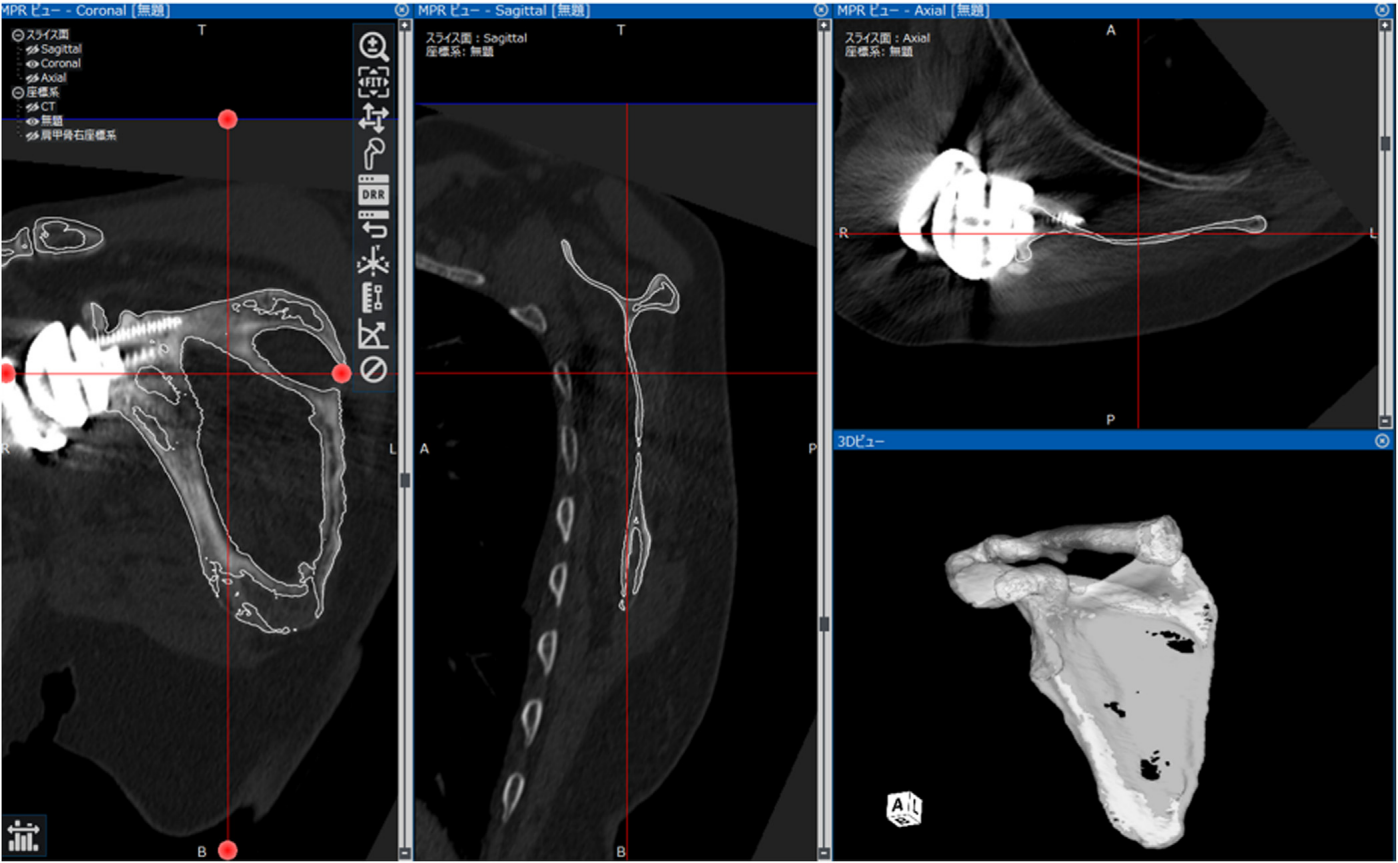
The method of superimposing postoperative CT and preoperative data. The coordinate system is constructed by superimposing the preoperative data on the postoperative CT images. *Red line*: lines to create multiplanar reconstruction; *White line*: preoperative data. *CT*, computed tomography.
